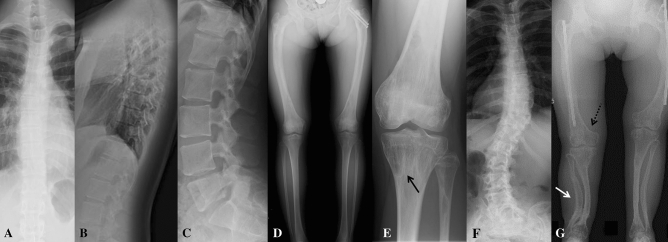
Skeletal radiographs of two patients with a novel heterozygous *IFITM5* mutation p.N48S. Images show (A) mild scoliosis, (B, C) normal thoracic and lumbar vertebral height without vertebral compression fractures, (D) mild femoral bowing with diaphyseal cortical thickening, and (E) metaphyseal osteopenia intermingled with sclerotic striations (arrow), cystic (tumor-like) lesions, and preserved cortical thickness of the distal femur and proximal tibia in an 18-year-old female; and (F) severe scoliosis and (G) long-bone deformity with metaphyseal widening (dashed arrow) and diaphyseal bowing (white arrow) in a 47-year-old female
